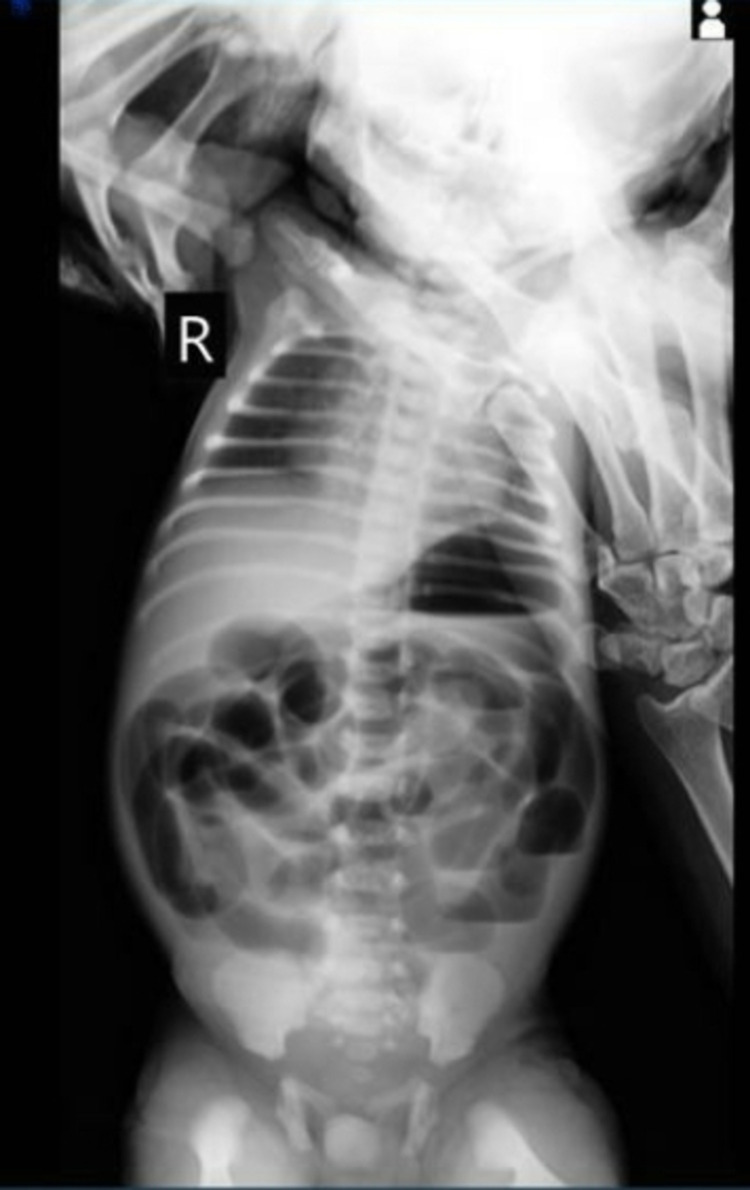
X-ray abdomen showing dilated loops of bowel and absence of bowel gas pattern.
